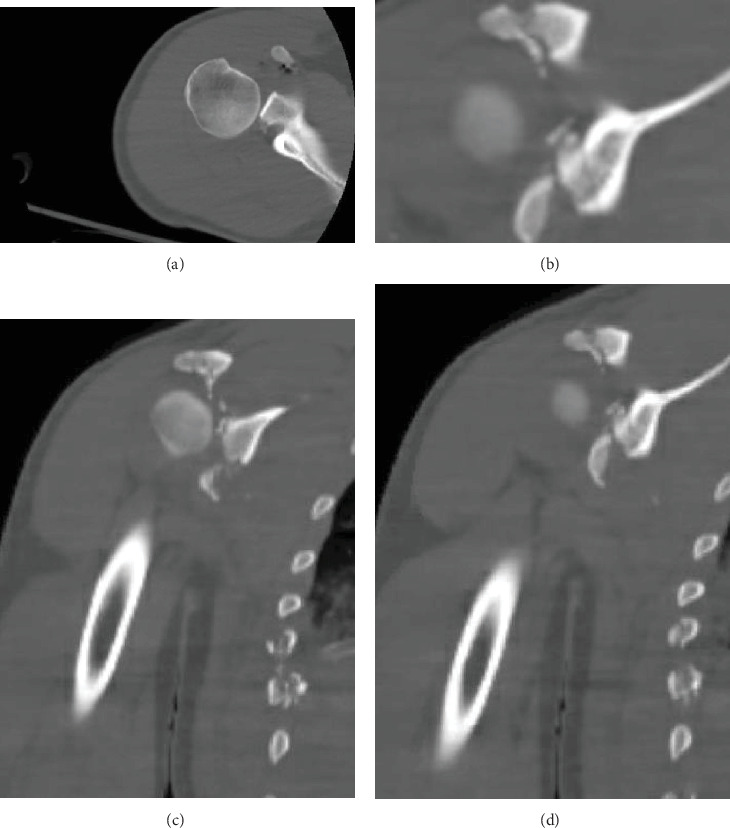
(a) Axial CT of the right shoulder. (b) Axial CT 2 of the right shoulder. (c) Coronal CT of the right shoulder. (d) Coronal CT 2 of the right shoulder.
